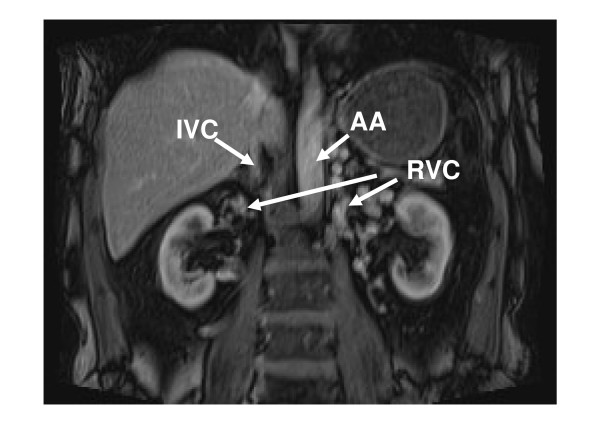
MRI showing renal vein collaterals (RVC) collecting venous blood return from both kidneys. AA, abdominal aorta; IVC, inferior vena cava
